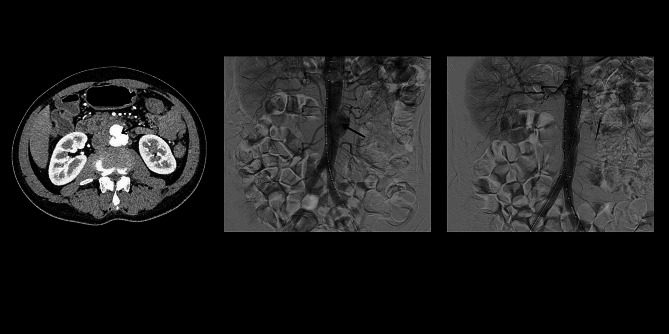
The left image depicts an infrarenal abdominal aortic pseudoaneurysm (1.7 cm × 3.4 cm) at the L2/3 level as seen on CTA. The middle image shows the pseudoaneurysm before endovascular treatment via angiography. The right image demonstrates the absence of contrast agent leakage peri-stent after the operation
